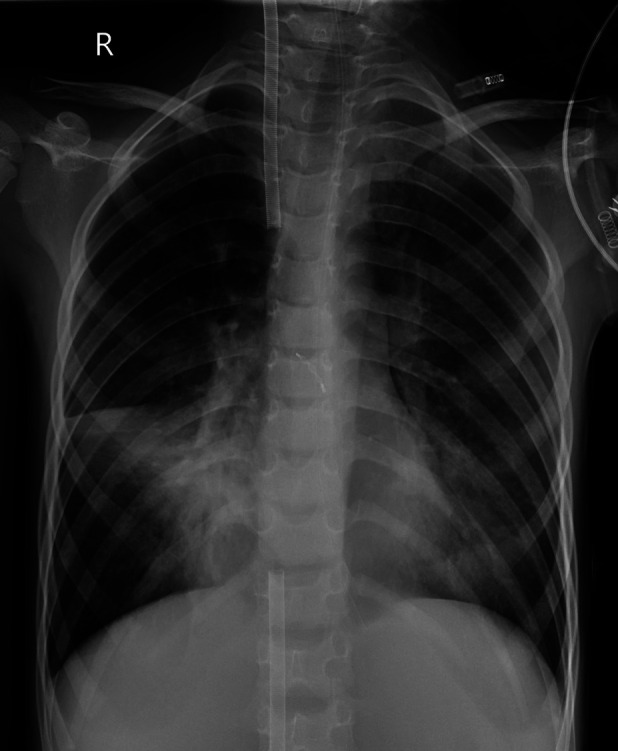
Chest x-ray performed 99 h after ECMO initiation.
